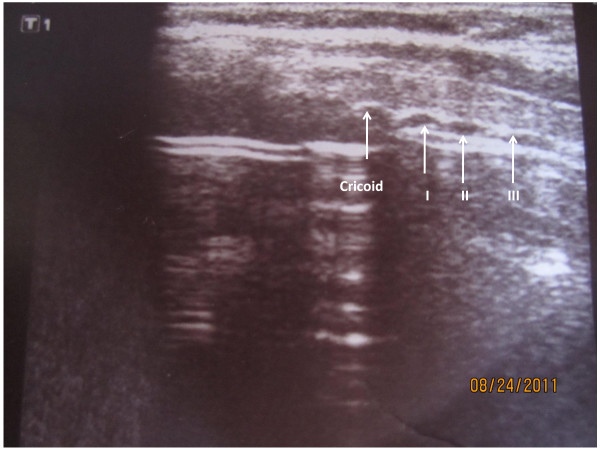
Ultrasound image of the trachea. Longitudinal view of the trachea shows the cricoid cartilage and the tracheal rings; important anatomical references to localize the site of puncture of the trachea. The endotracheal tube has been pulled back. I, II, III (first, second, and third tracheal rings); image obtained with an 8 MHz vascular probe.
